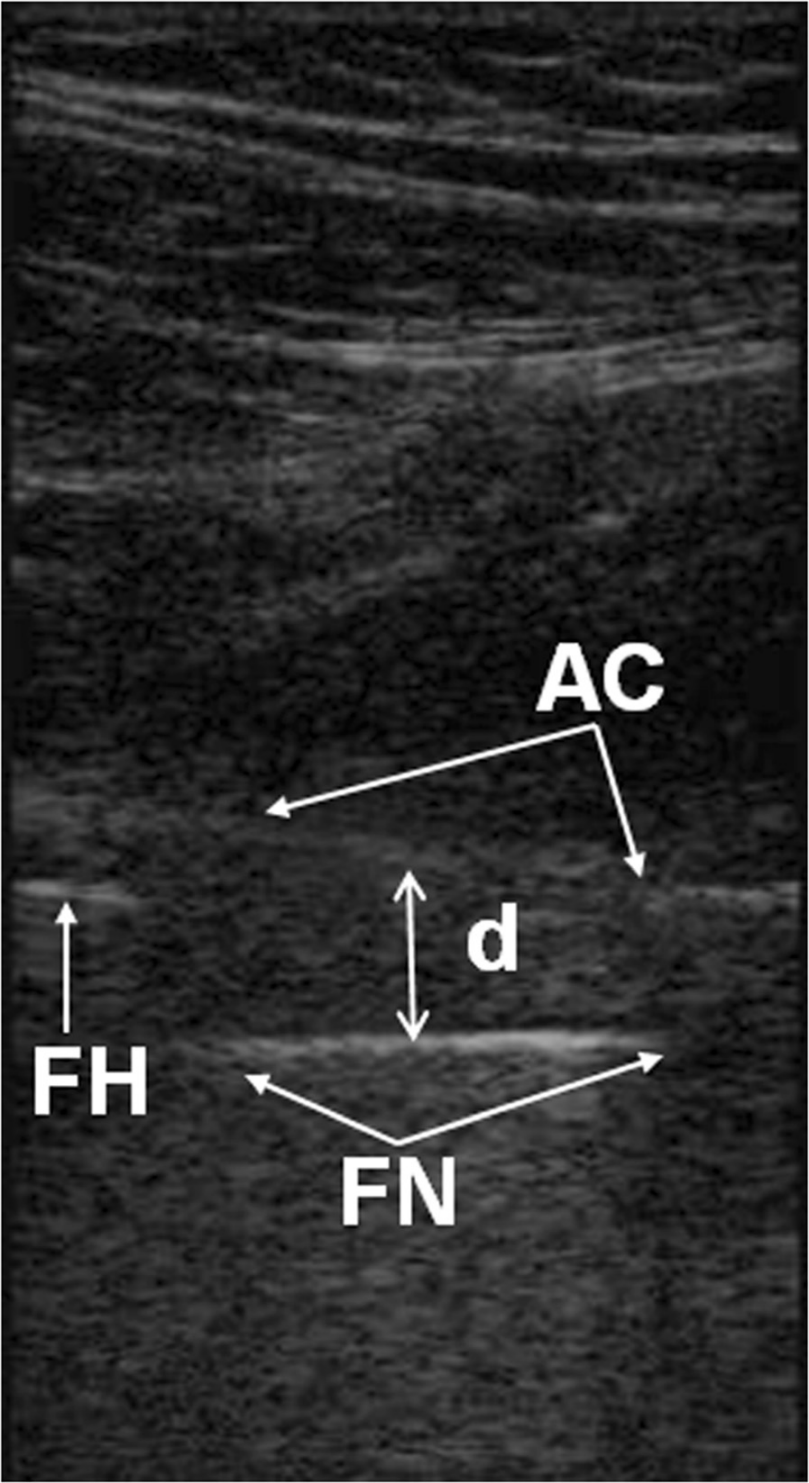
Sonographic measurement of the capsular distance in THA. AC - echo from the anterior surface of the anterior hip joint capsule. FN - echo from the anterior surface of the prosthetic femoral neck. FH – echo from the anterior surface of the prosthetic femoral head. d - represents the distance between anterior capsule and prosthetic femoral neck.
